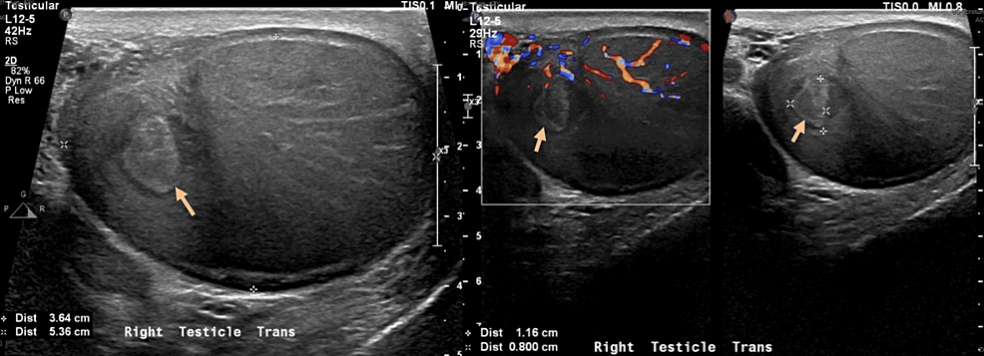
Shows right testicular ultrasonography and doppler ultrasound demonstrating a heterogeneous intratesticular lesion with relatively low echogenicity.
